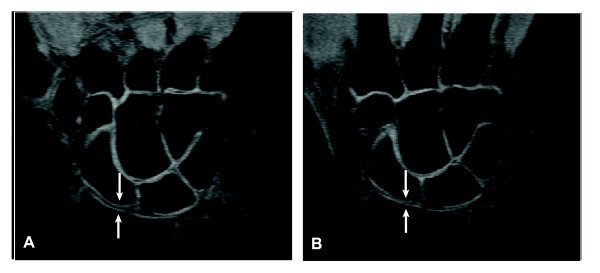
Assessing cartilage directly is more accurate than measuring joint-space width. Intact articular cartilage can be seen in these magnetic resonance images of the wrist as bands of high-signal-intensity tissue lining the surfaces of the bones and showing sharp contrast with low-signal-intensity joint fluid along the articular surfaces, and very low-signal-intensity bone cortex and suppressed marrow fat along the subchondral surfaces. Follow-up image (B) shows narrowing of the radioscaphoid joint space (arrows) relative to that in image (A). However, this narrowing is the result of displacement of joint fluid from between the intact articular cartilage surfaces because of abduction of the wrist rather than because of thinning of the cartilage plates themselves. Thus, joint-space width can be an inaccurate measure of cartilage thickness.
